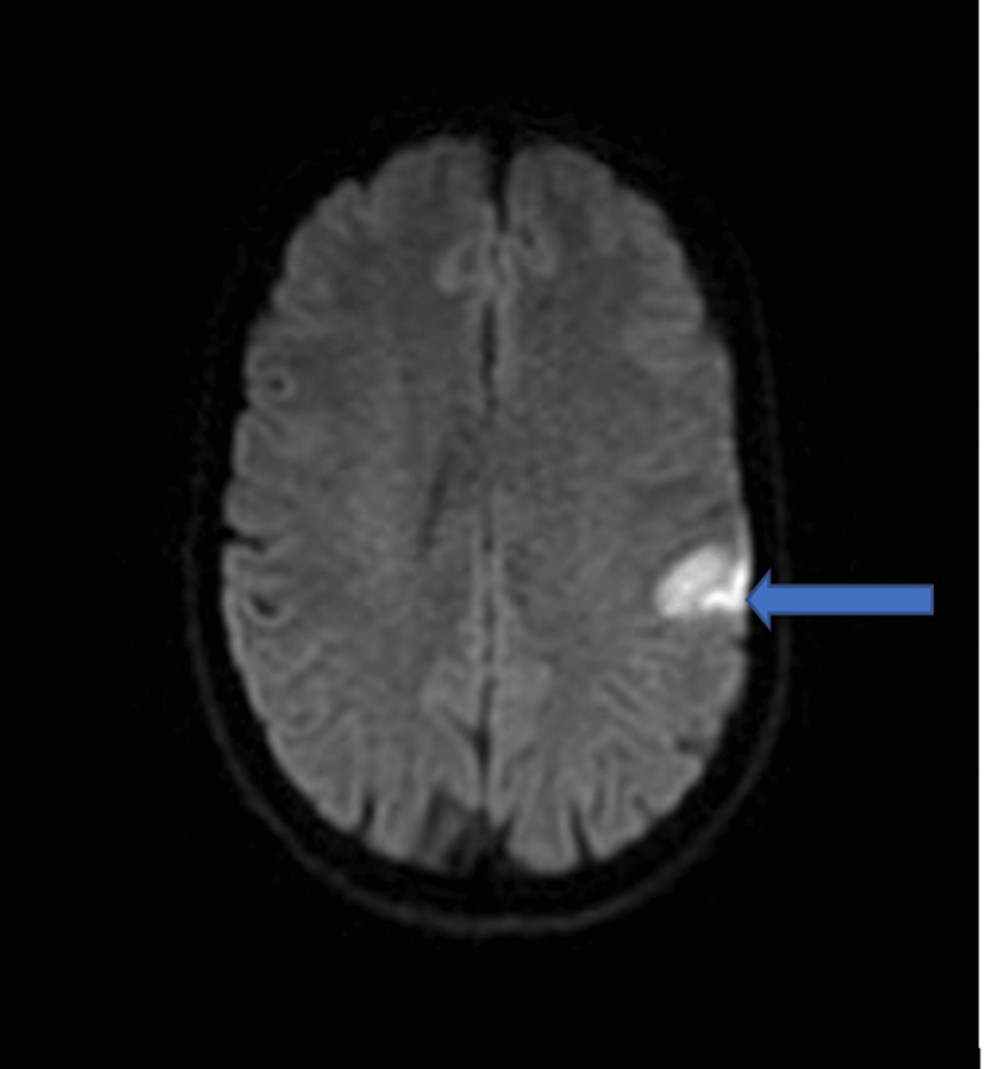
MRI DWI image showing hyperintensity in the left frontoparietal cortex (blue arrow) DWI: diffusion-weighted imaging
